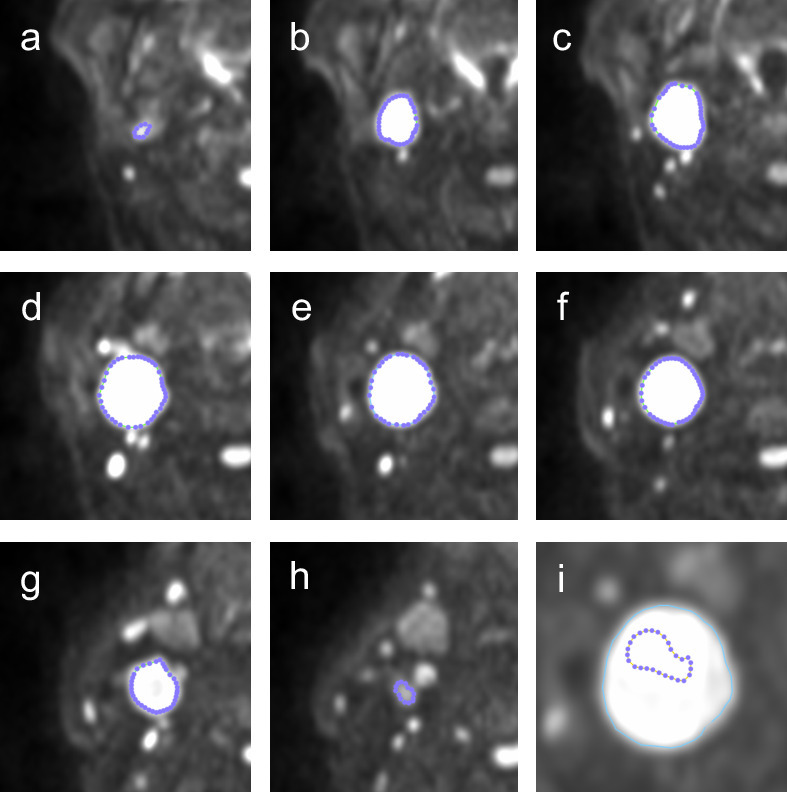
Volumetric ROI (ROI_v_) of the largest lymph node on the b800 image disease was manually delineated (a–h) on the b800 DWI sequence. ROI_a_ was analysed on slice e. i is a magnified view of the node in panel e with an example of a representative ROI (ROI_r_). This was focused on an area of increased DWI signal on the b800 image but excluded any areas of necrosis.
